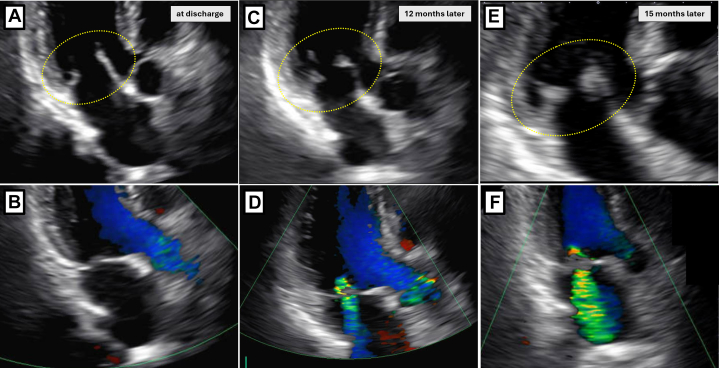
Progression of Mitral Valve Vegetations on TTE (A and B) At discharge, no vegetations were observed on the mitral leaflets, and no mitral regurgitation was present. (C and D) At 12 months after discharge, thickening of the mitral valve leaflets appeared, accompanied by mild-to-moderate mitral regurgitation. (E and F) At 15 months after discharge, the vegetations had enlarged, and severe mitral regurgitation developed. TTE = transthoracic echocardiography.
